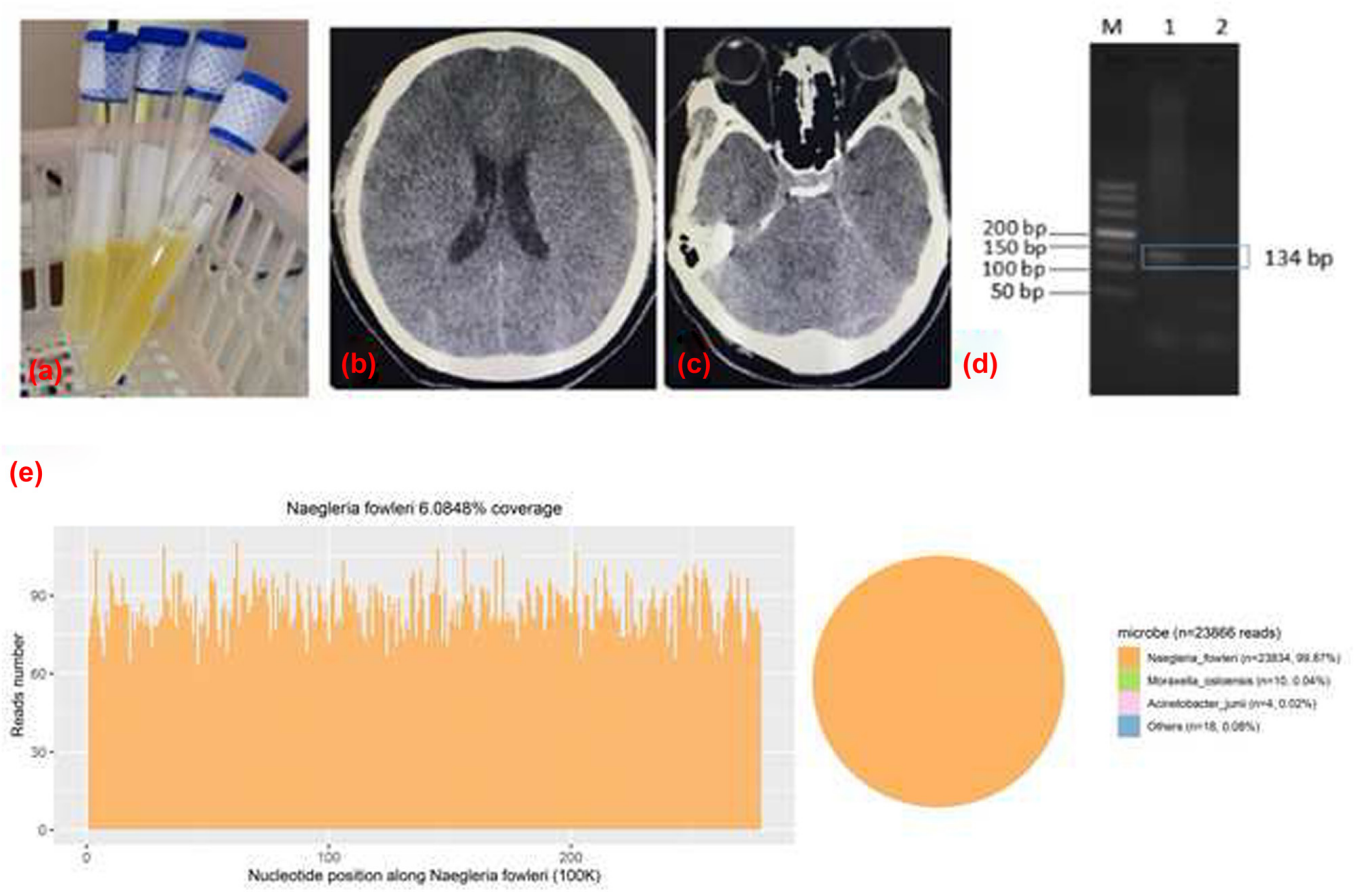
(a) After admission, there showed a cloudy and yellowish CSF. (b and c) Head CT scan showing the decreased density of brain parenchyma, and the narrowed cistern and sulcus, which indicate diffuse swelling of the brain. (d) The physical fragment of *N. fowleri* was 134 bp. The results were consistent with the electrophoretic bands of the patient (Lane 1), and the negative control (Lane 2) had no bands. It is indicated that *Naegleria fowleri* exists in this sample. (e) mNGS results show that a total of 23,834 specific reads of *N. fowleri* were detected in CSF, the coverage was 6.08%.
